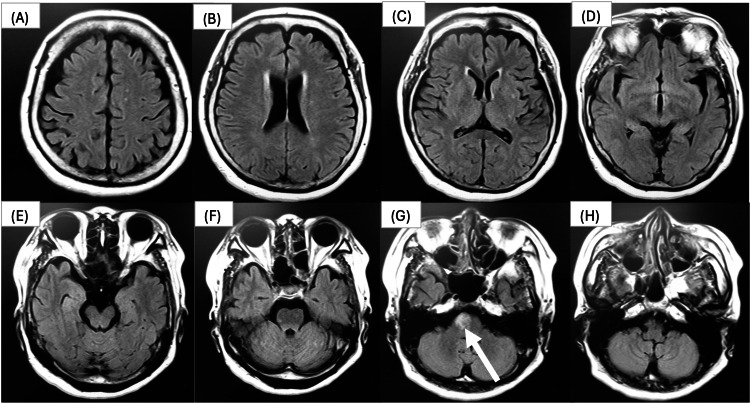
Five days after onset, T2 FLAIR image. (A, B, C, D, E, F, H) Brain images by cross-section. (G) High signal intensity observed in the pons. FLAIR: Fluid-Attenuated Inversion Recovery.
